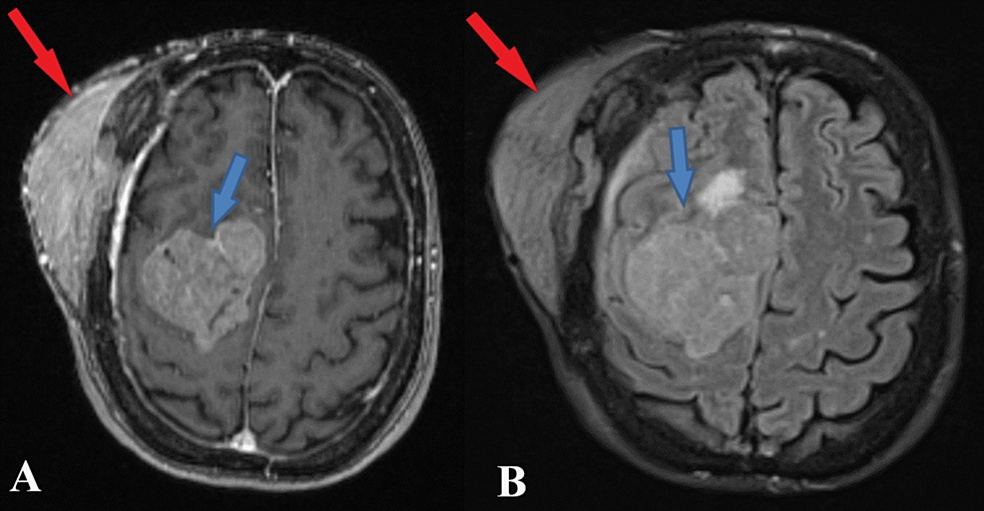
Preoperative MRI T1 and T2 sequence The axial section of T1 sequence (A) and axial section of T2 sequence (B) both depict a giant intracranial (blue arrows) and extracranial (red arrows) meningioma with significant osteolysis of the skull after extensive extracranial invasion.
